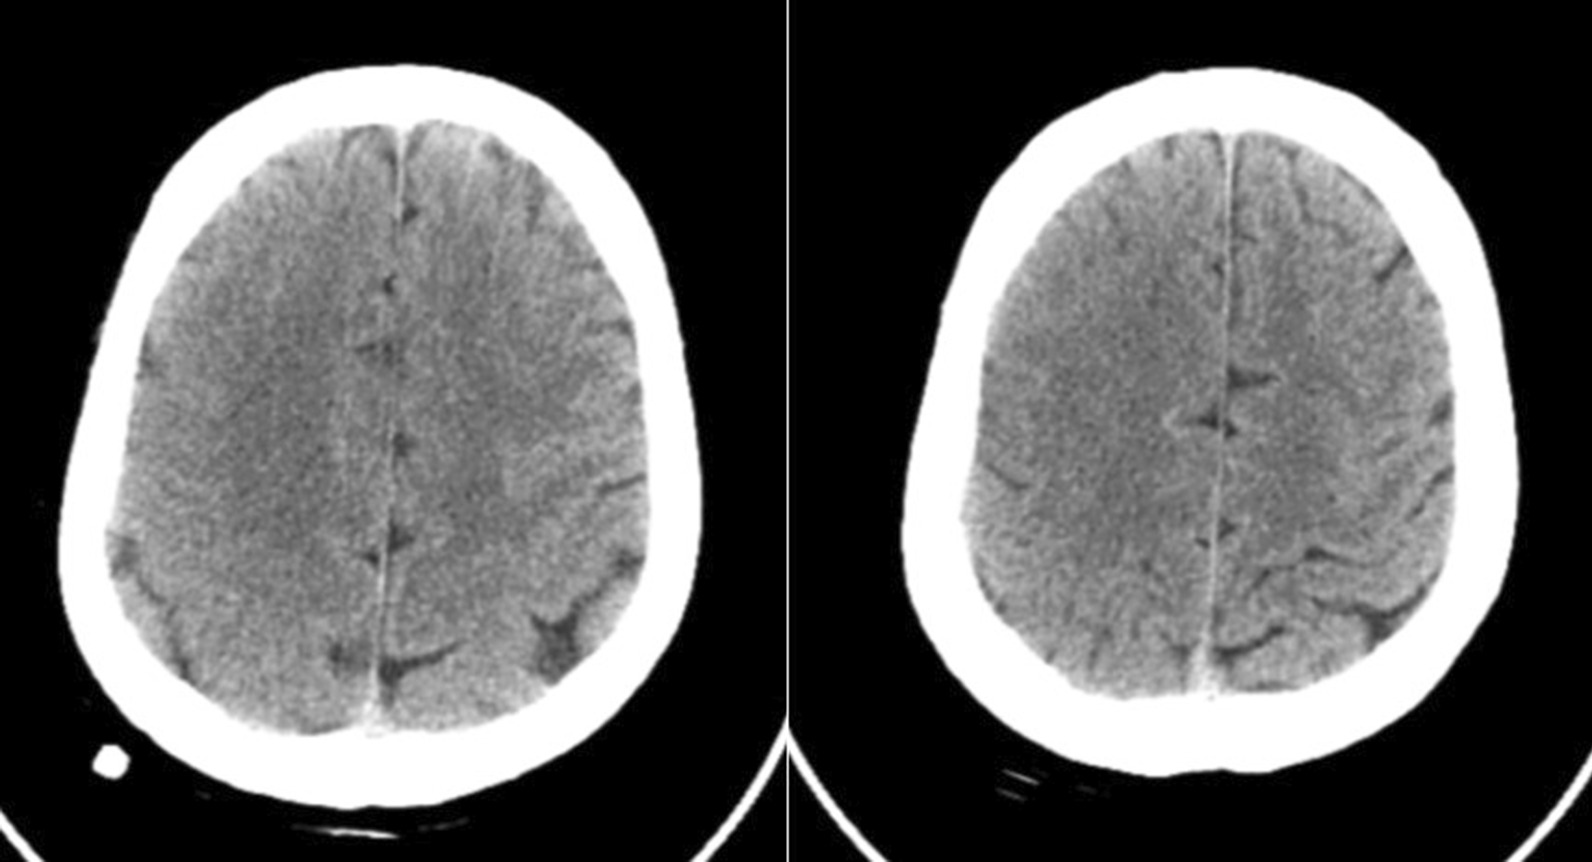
The repeated cerebral CT scan performed 24 h after bronchoscopy demonstrated no signs of air embolism
